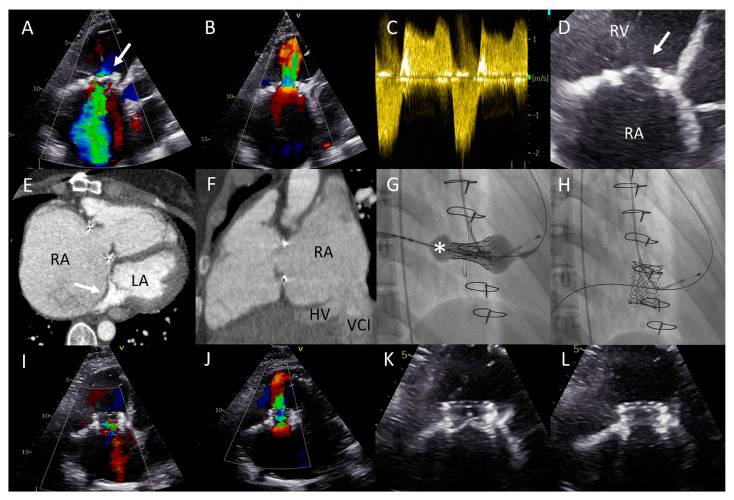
(A) Color Doppler apical four-chamber view shows severe tricuspid regurgitation (TR) with a wide vena contracta (arrow) and a systolic jet reaching the roof of the enlarged right atrium (RA). (B) Color Doppler showing turbulent inflow through the tricuspid valve (TV) bioprosthesis and aliasing, mean gradient was elevated at 4–5 mmHg. (C) Continuous wave Doppler showing a dense TR signal with low velocity. (D) Calcified and degenerated tricuspid bioprosthesis (arrow). (E) Axial slice through a computed tomography (CT) scan at the level of the right ventricle shows a giant RA and an intra-atrial septum deviation towards the left atrium (arrow), partially suppressing it. (F) Sagittal CT slice shows the inflow angle of the inferior vena cava-right RA and the RA-TV. Note the distended hepatic vein (HV). (G,H) Right anterior oblique fluoroscopy projections show the expansion of the Sapien 3 valve (asterisk) using the ring of the degenerated bioprosthesis as the reference and the final result, respectively. (I,J) Apical four-chamber color Doppler views showing normal function of the valve-in-valve bioprosthesis (appreciate the difference with (A,B), respectively). (K,L) View of the valve-in-valve bioprosthesis in systole (closed) and diastole (open), respectively.
